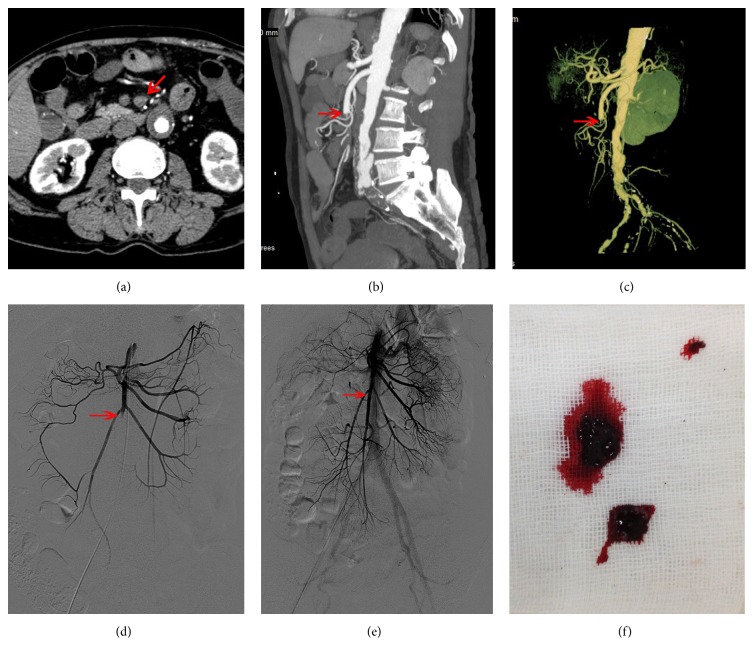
A 41-year-old female patient (Case (9) in Table 1) with acute thromboembolic occlusion of the superior mesenteric artery presented with acute-onset abdominal pain beginning 12 hours before the admission. ((a)–(c)) Computed tomography angiography of the abdomen indicated abrupt occlusion of the superior mesenteric artery (SMA) (arrow). (a) Cross-sectional image; (b) sagittal image; (c) three-dimensional lateral image; ((d), (e)) digital subtraction angiography (DSA) demonstrating the occlusion of the SMA before the treatment ((d), arrow) and complete restoration of the flow in SMA after the aspiration and thrombolysis ((e), arrow); (f) aspirated thromboemboli that were observed. Arrows indicate the morphological characteristics of artery.
